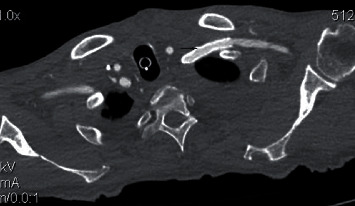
Postoperative CTA showed the proper position of the chimney graft inside the LSA (arrow) and the patency of the vessel.
